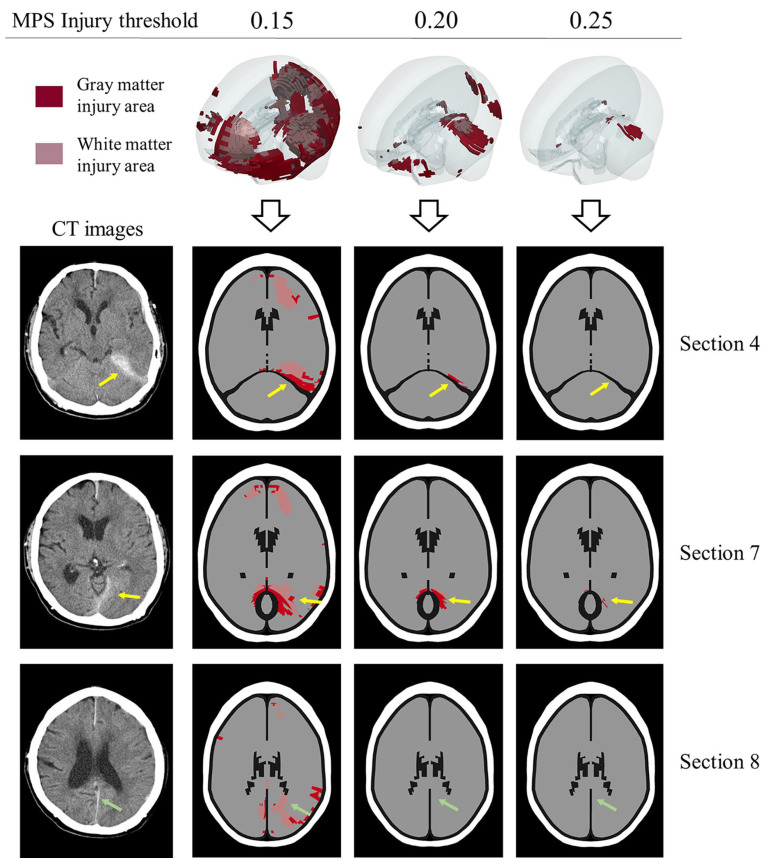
The rationality of the MPS objective injury threshold (0.2) in predicting entire brain injury is evaluated. The prediction effects of thresholds 0.15, 0.2, and 0.25 are compared with CT images. Red color indicates gray matter element injury, pink color indicates white matter element injury, and elements not highlighted are not injured. The yellow arrow and green arrow indicate the injuries in the supra-tentorium cerebelli and between the cerebral hemispheres, respectively. The prediction area of 0.15 is significantly larger, and the prediction area of 0.25 is significantly smaller. In comparison, the prediction effect of 0.2 is more consistent with the CT images.
